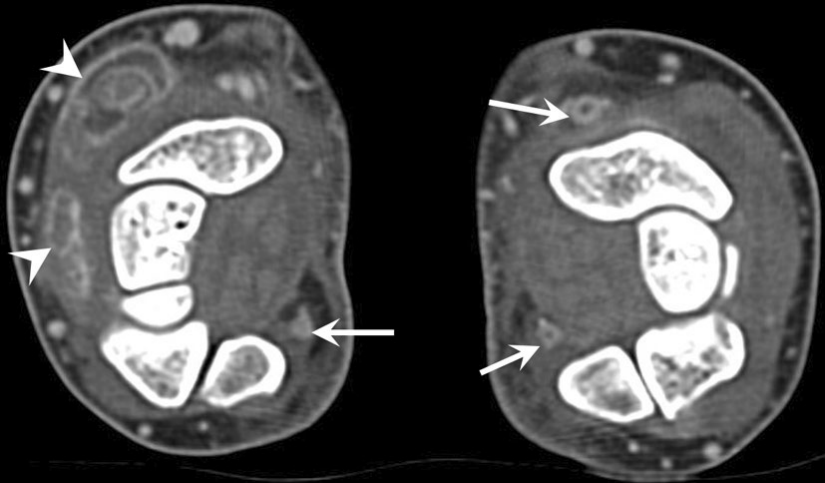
Buerger’s disease in a heavy smoker 41-year-old woman presenting with ischaemic ulcers of the fingers and arthralgia. Bilateral CTA shows occlusion and wall thickening of the right RA and of both UA (straight arrows). Note also a tenosynovitis of the extensor carpi radialis and of the extensor digitorum of the right wrist (arrowheads)
